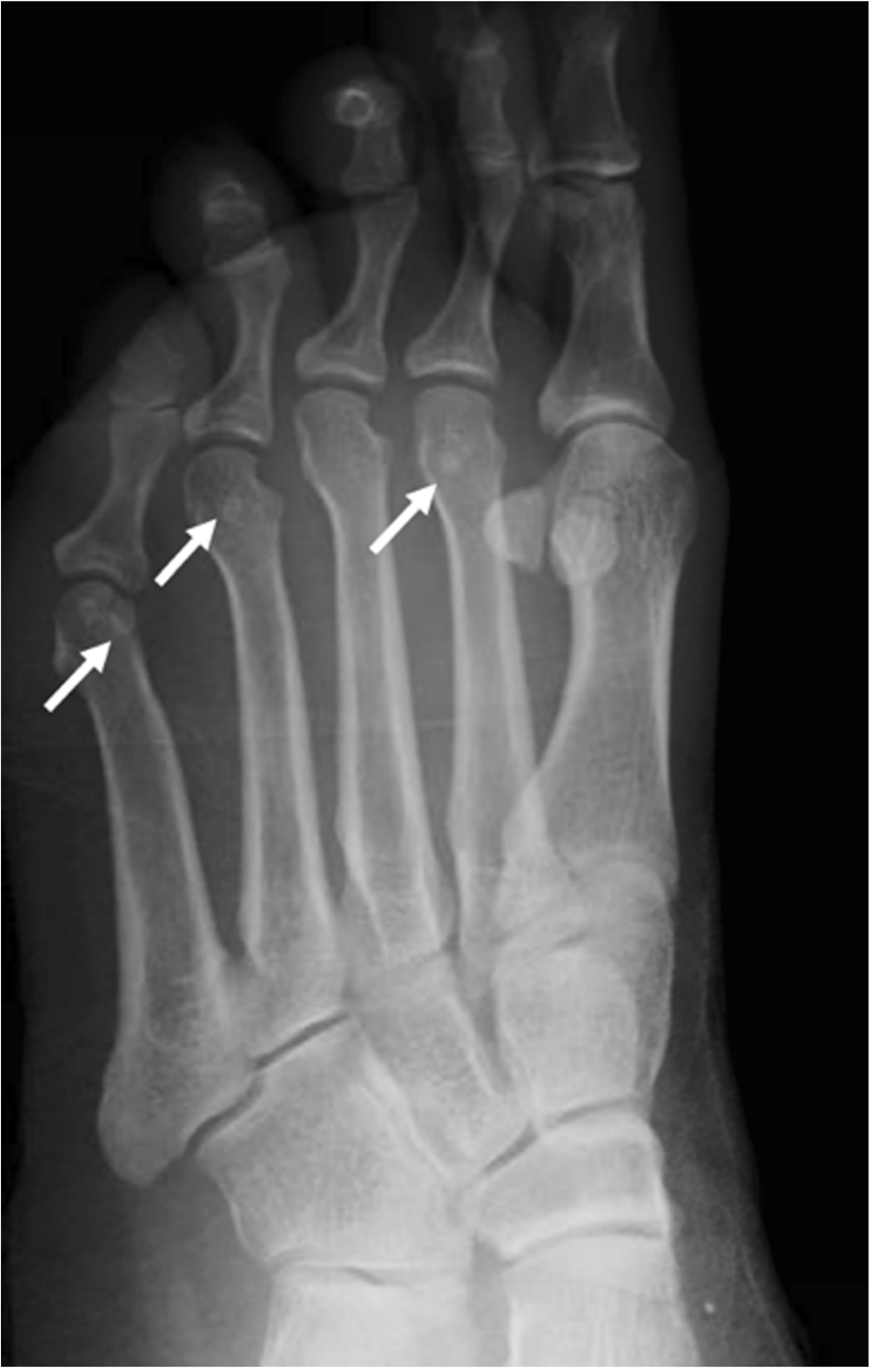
Lesser metatarsal sesamoids. Oblique radiograph on a 42-year-old woman, referred after trauma of the ankle. Incidental finding of multiple lesser sesamoids in the fifth, fourth, and second metatarso-phalangeal joints (white arrows). The fifth is the most frequently found one. Lesser sesamoid pathology is very rare
